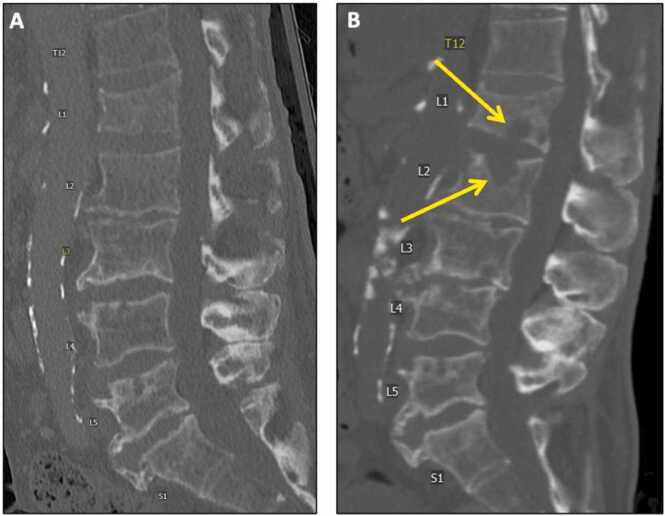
Discitis-osteomyelitis changes on CT. Comparing to prior CT L-spine from March 2022 (A), a CT L-spine from August 2022 (B) shows new prominent erosive endplate changes at L1-L2 (arrows) and some erosion at L3-L4, concerning for discitis-osteomyelitis.
